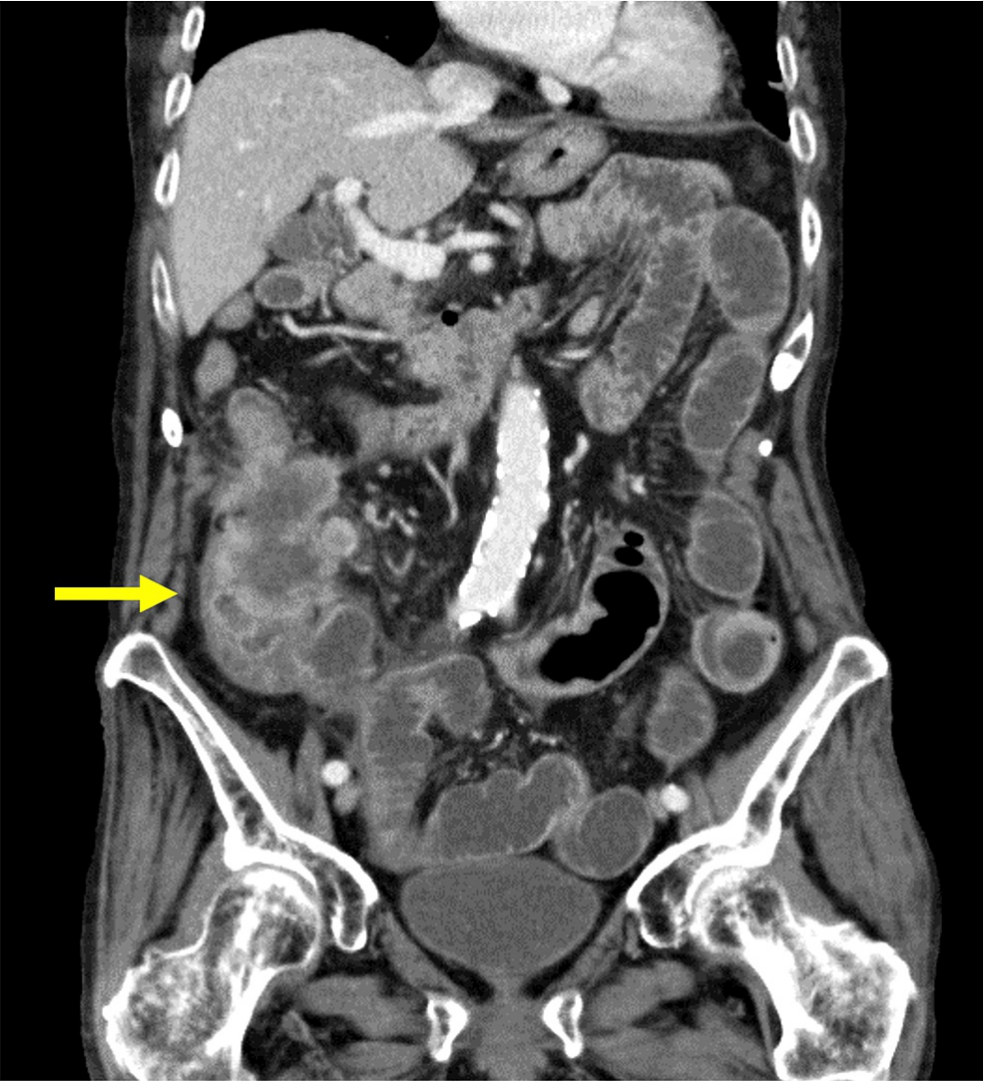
Preoperative abdominal contrast-enhanced CT Abdominal contrast-enhanced CT revealed massive colon cancer with invasion of the terminal ileum (yellow arrow) and bowel obstruction.
